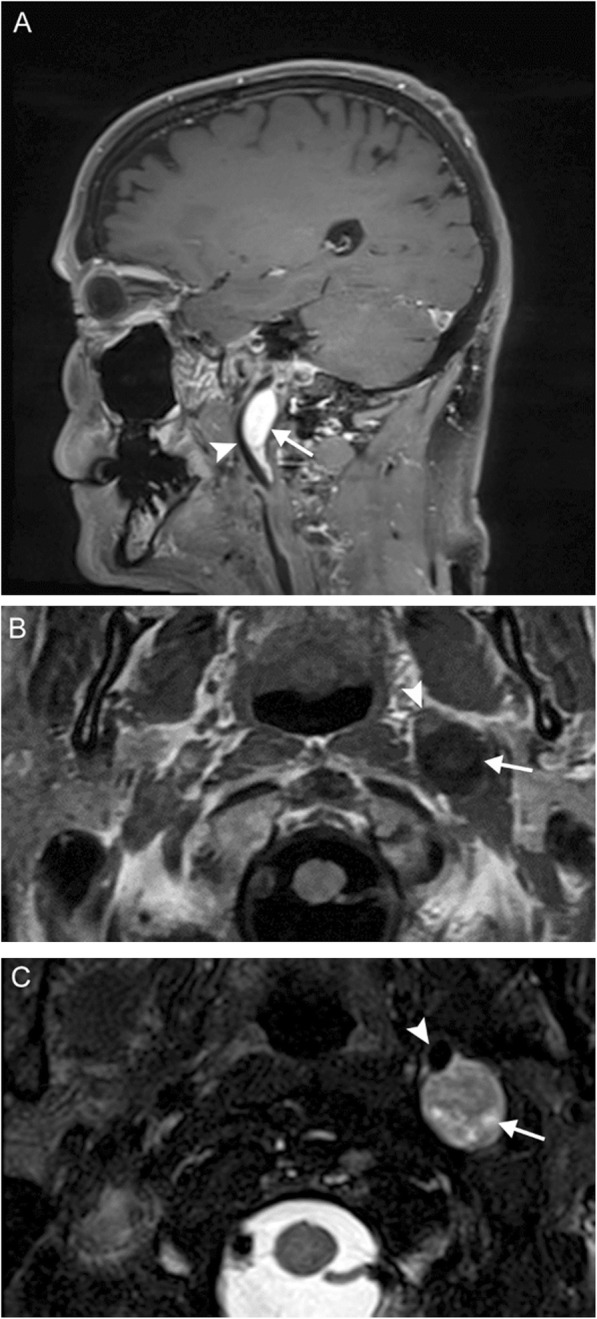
Vagal paraganglioma. A 67-year-old man presenting with raspy voice. Sagittal T1 post-contrast (a), axial T1 (b), and axial T2 (c) MR images reveal a T1-hypointense, avidly enhancing lesion with heterogeneous areas of high T2 signal (arrows) centered within the left carotid space. The internal carotid artery is displaced anteromedially (arrowheads). Pathology was consistent with a vagal paraganglioma (glomus vagale tumor)
